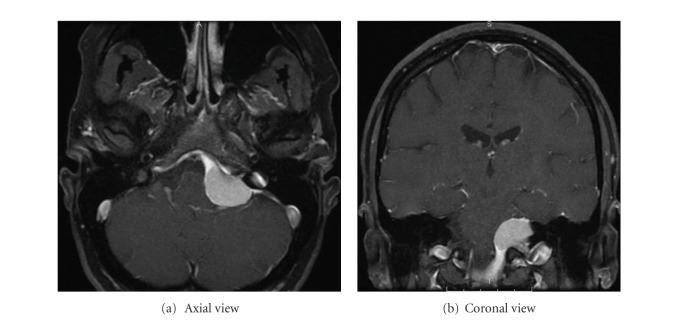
Preoperative axial and coronal MRI images of the patient's brain. Gadolinium enhancement demonstrates a large mass in the left cerebello-pontine angle.
